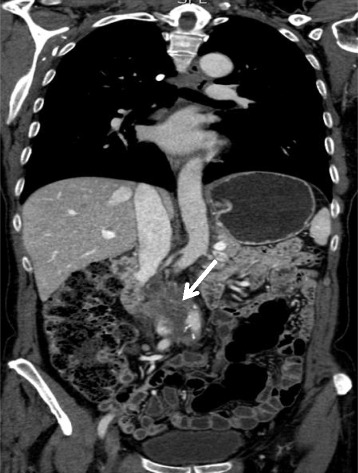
CT scan image. Coronal view showing retroperitoneal heterogeneous soft tissue density mass that compresses and thrombosis the infrarenal IVC of 8 × 4.6 cm in size (white arrow).
